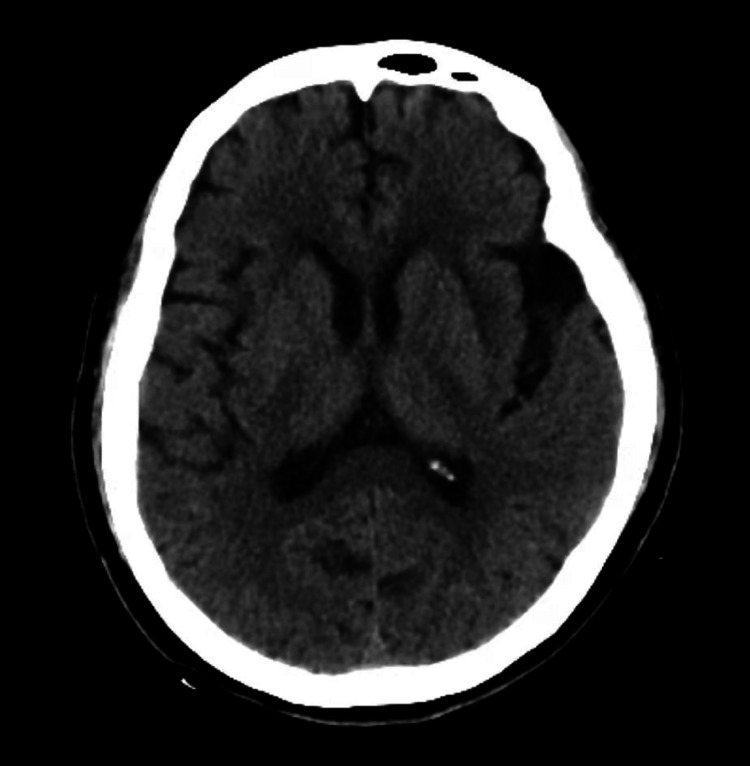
Axial CT scan of the head without contrast showing brain atrophy and leukoaraiosis. CT: computed tomography
